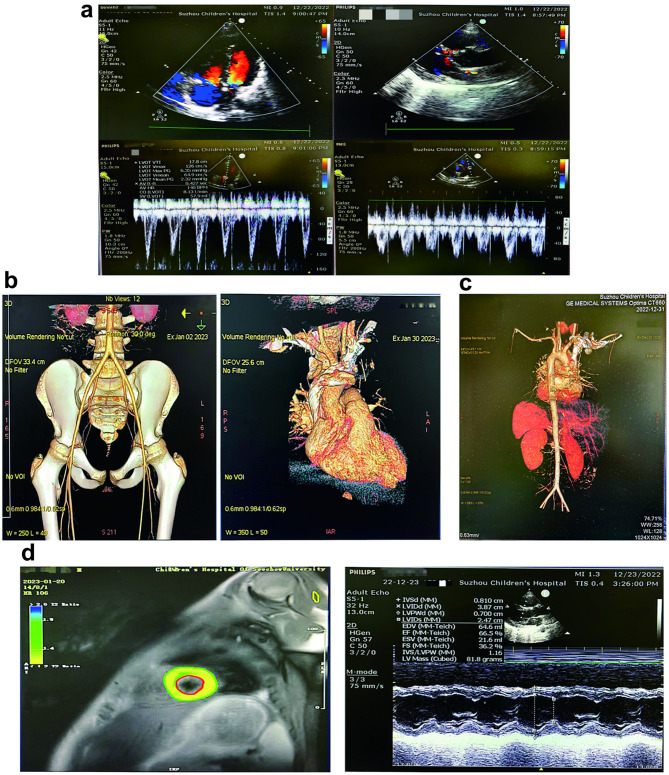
Selected imaging of the child’s initial admission to the hospital. (a) Cardiac ultrasound showed a 40 mm × 18 mm medium-high echogenic mass in the right ventricular cavity, with clear boundaries and irregular morphology, oscillating with the heartbeat, and a right ventricular free wall thickness of 3.9 mm. Pulmonary artery pressure was elevated, with mild tricuspid regurgitation and a pressure difference of 33mmHg. LVOT VTI: 21.1 cm, SV:55.1 ml, CO:6.62 L/min. (b) CT of the lower limbs showed marked thickening of the common iliac vein to the bilateral femoral veins with uneven density. PW measurement showed blood flow velocity in the common femoral vein Vmax = 0.09 m/s. (c) (d) CTA showed multiple emboli in both pulmonary arteries, posterior superior vena cava and right ventricular filling defects, and large, clumpy hyperdense shadows in the lower lobes of both lungs. (d) There were effusions in the bilateral thoracic and pericardial cavities
